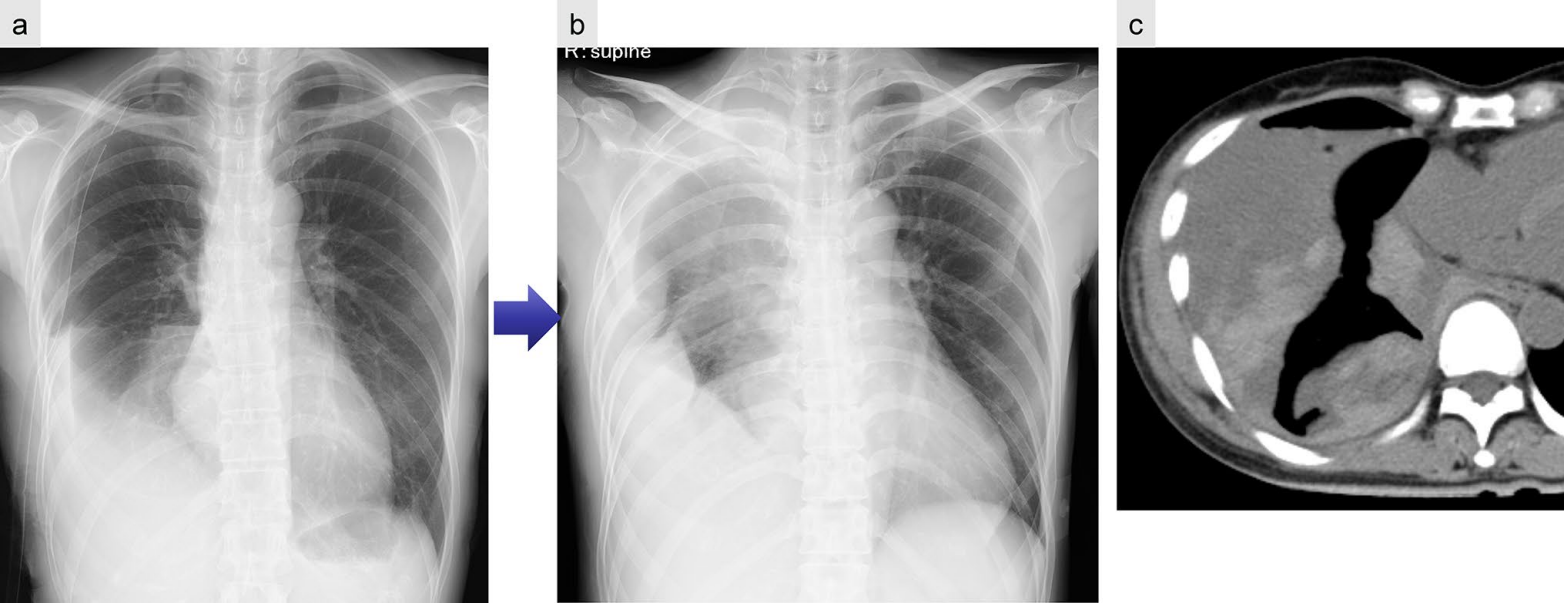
Hemothorax after right lower lobectomy. The patient developed hemorrhagic shock following the removal of the drainage tube. CXR after tube removal demonstrated a sudden decrease in tight-sided translucency (a, b). Urgent non-contrast CT revealed a pleural effusion containing high-attenuation areas, indicative of hemorrhagic fluid (c)
